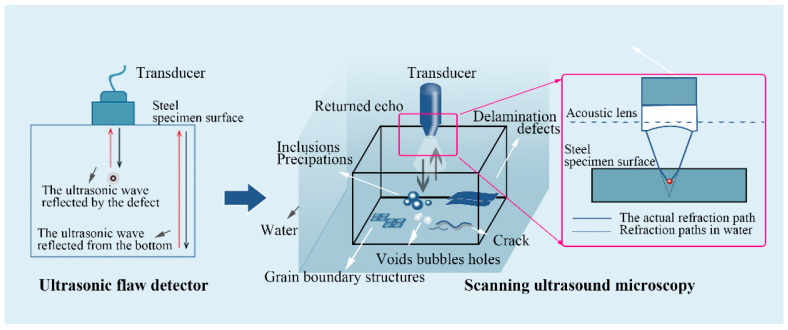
Testing principle of the ultrasonic instrument.
